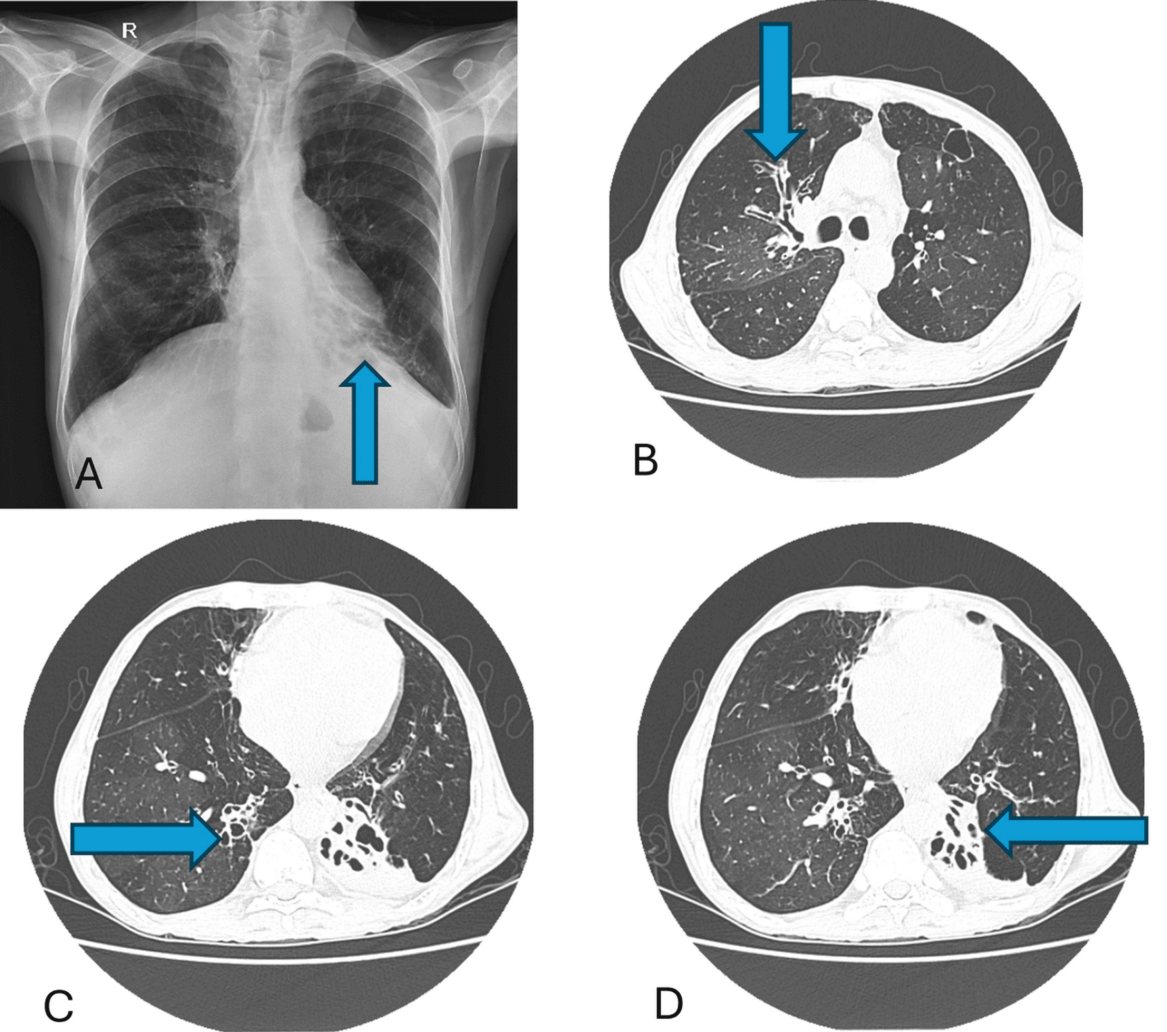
A. Chest X-ray PA view; B, C, and D. CT chest axial view Computed tomography (CT) of the chest shows bilateral bronchiectasis with mild bronchial wall thickening in the right lung and left upper lobe. It also shows left lower lobe collapse with bronchiectasis. *Blue arrows indicate bronchiectasis.*
